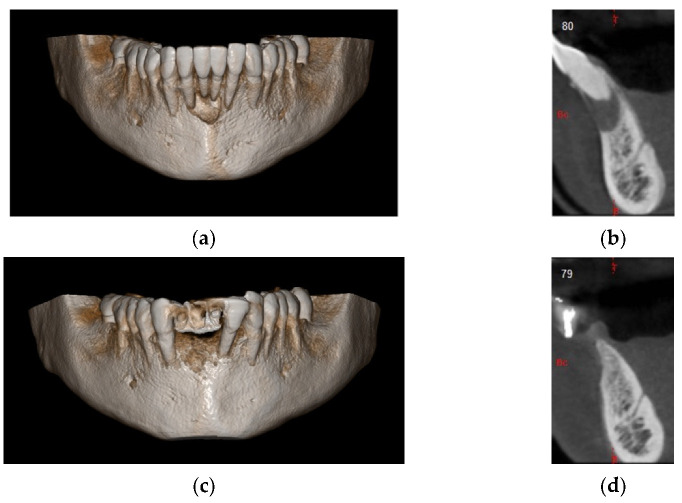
(a) Initial 3D situation; (b) CBCT of the initial situation; (c) Final CBCT post 3D regeneration after 4 months; (d) X-ray of the situation post regeneration after 4 months.
